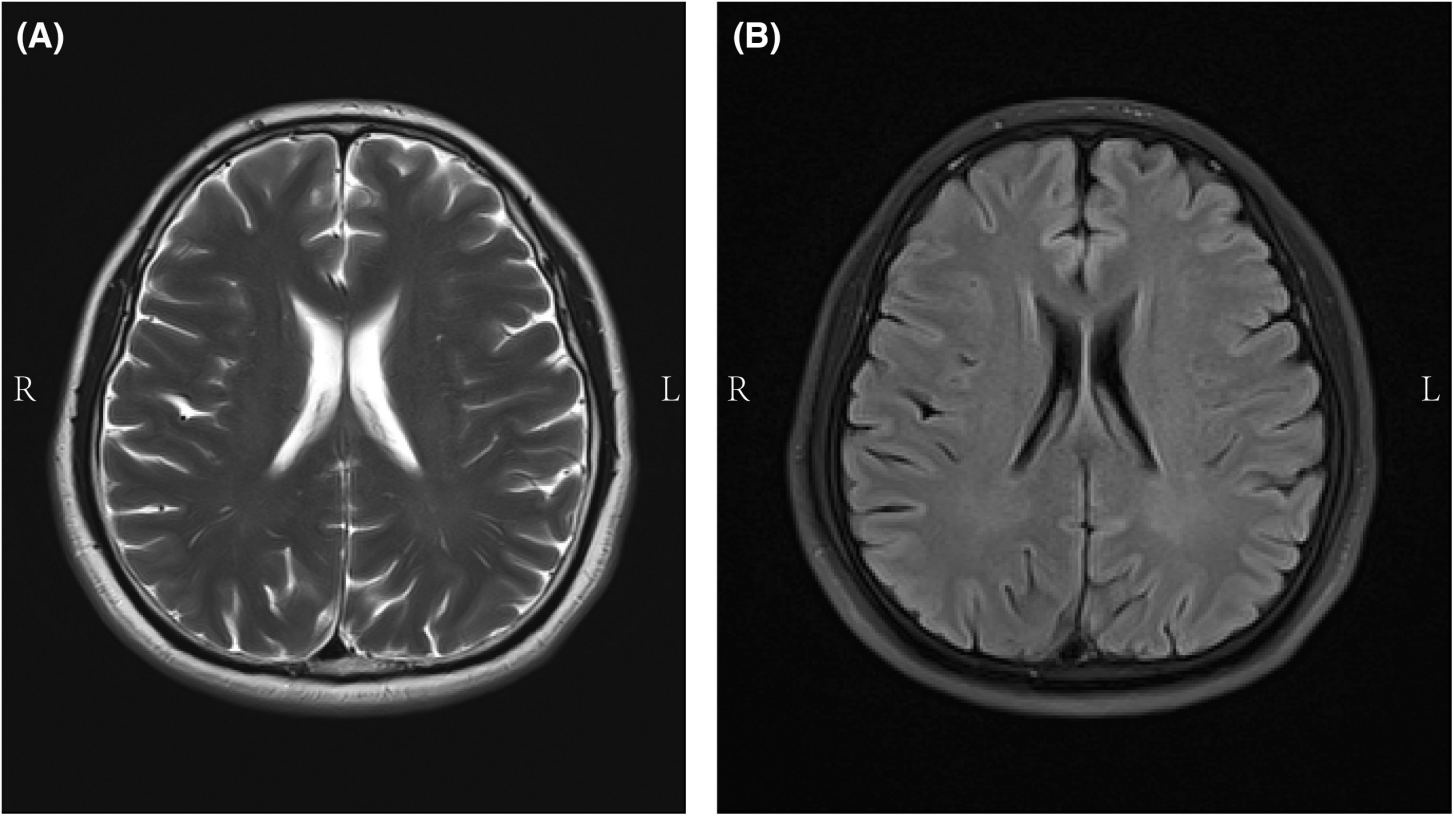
Brain MRI. Axial section of the lateral ventricle shows no obvious abnormalities. (A) T2‐weighted image (T2WI); (B) T1‐weighted image (T1WI). L, left; MRI, magnetic resonance imaging; R, right.
